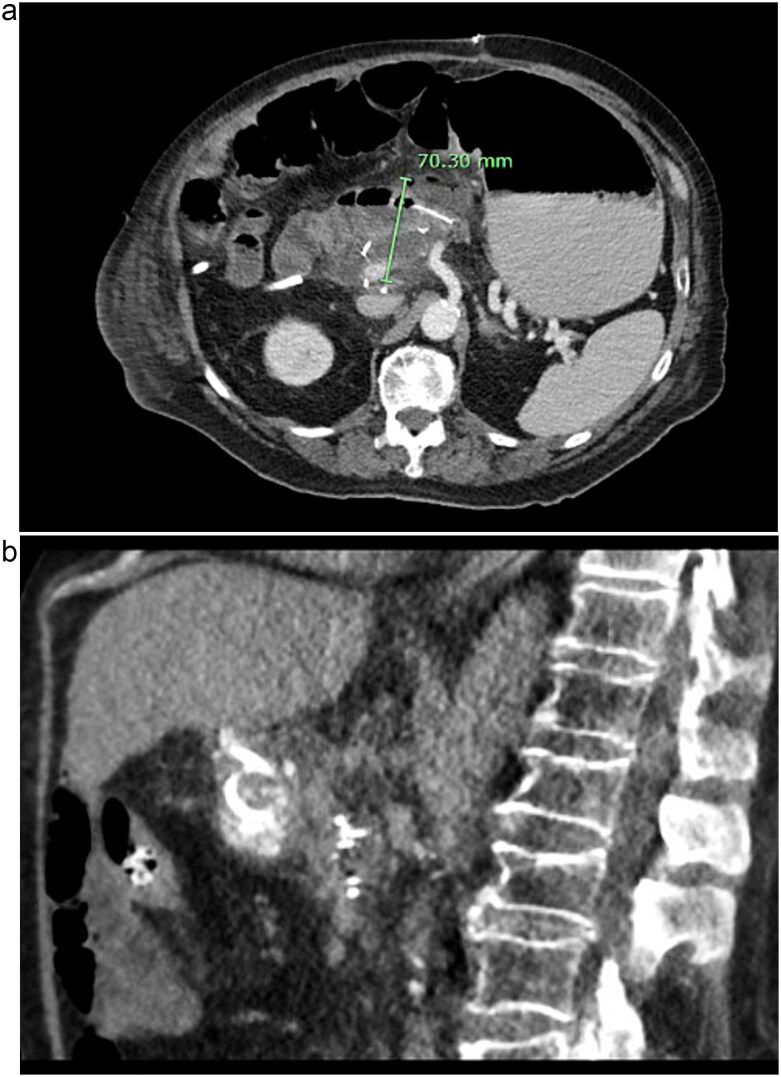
CT images of this patient’s post-operative pancreatic fistula (a) prior to IR guided intervention; (b) after percutaneous jejunostomy that was confirmed to rest within the blind jejunal end of the afferent loop after pancreaticoduodenectomy.
